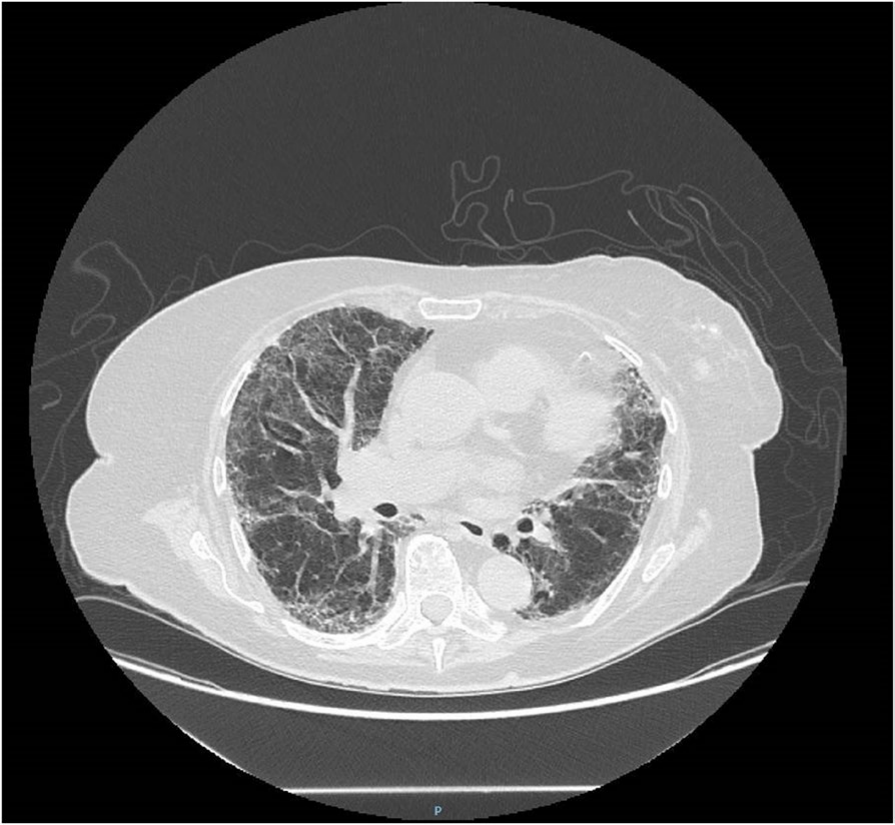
The second HRCT with severe and bilateral Sars Cov2 interstitial pneumonia
